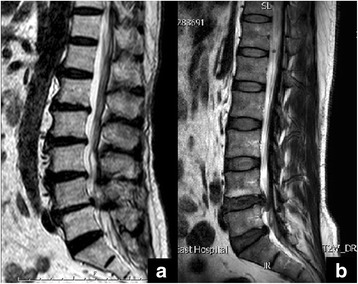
Lumbar MRI imaging of two patients who are both non-smokers and have neither alcohol addiction nor HBP history. a A 57-year-old patient with T2D for 12 years visiting our department because of low back pain for 3 years. Average HA1c during the recent 1 year is 7.2%. BMI is 19.81 kg/m^2^. The Pfirrmann scores from L1/2 to L5/S1 are all 5. b A 55-year-old patient without DM visiting our department because of low back pain for 3 years. BMI is 19.73 kg/m^2^. The Pfirrmann scores from L1/2 to L3/4 are 3 and from L4/5 to L5/S1 are 4
